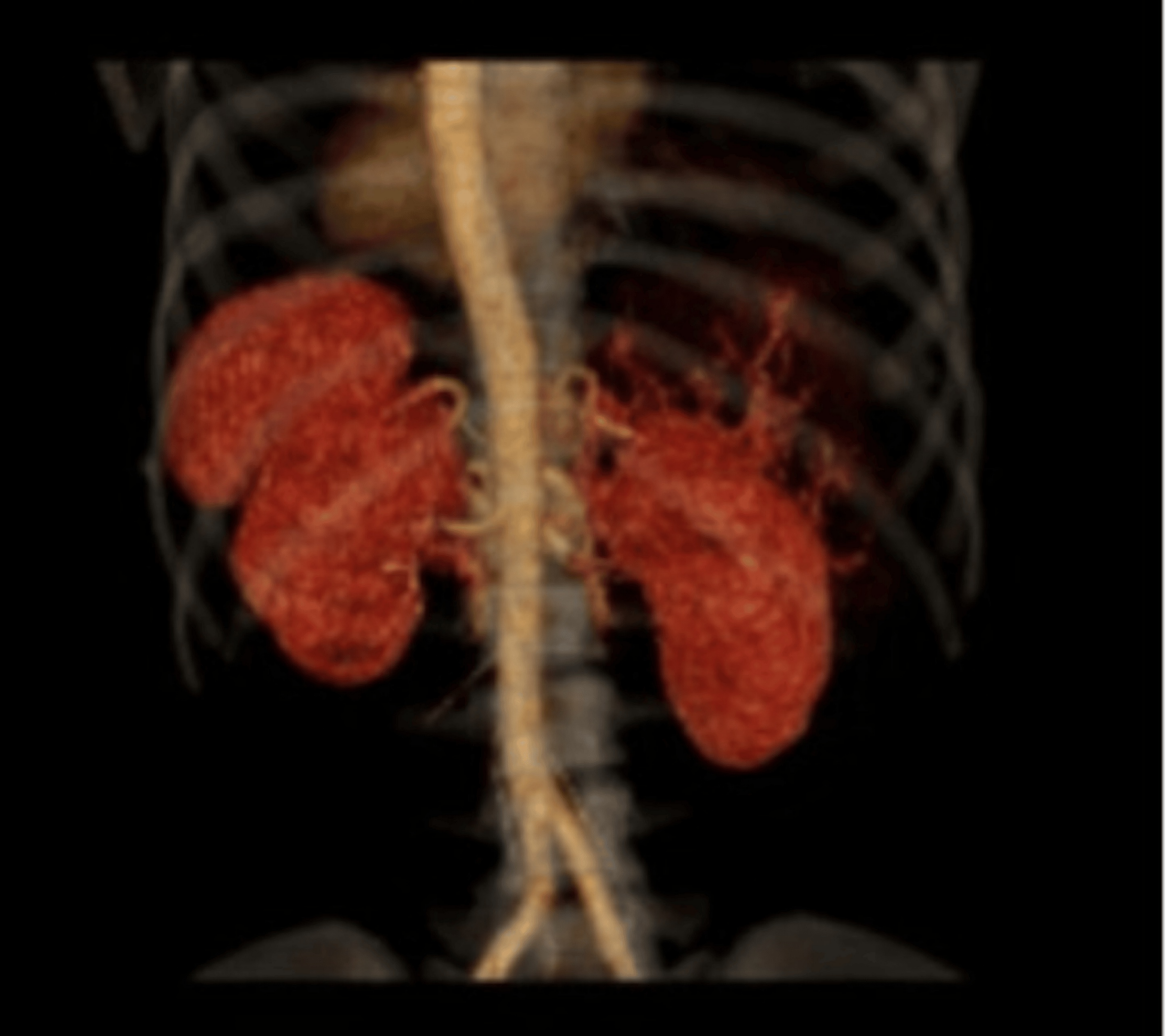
Three-dimensional reconstruction of the liver performed one week after admission Showing bilateral subcapsular hematomas (right lobe ≈1550 cc, left lobe ≈131 cc). Visualization generated with a Philips Brilliance 64 CT scanner (Koninklijke Philips N.V., Amsterdam, Netherlands). No new lesions or signs of active bleeding were observed.
